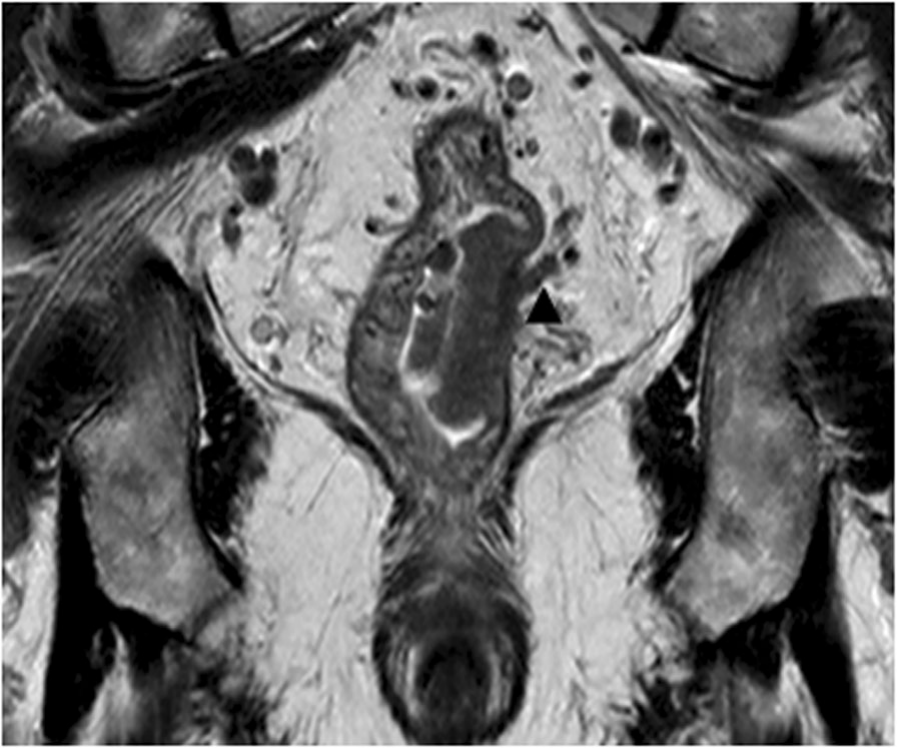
Extramural vascular invasion (EMVI) involvement. Coronal T2-weighted imaging showing focal expansion of the small perirectal vessel with intermediate signal intensity (black arrow head)
